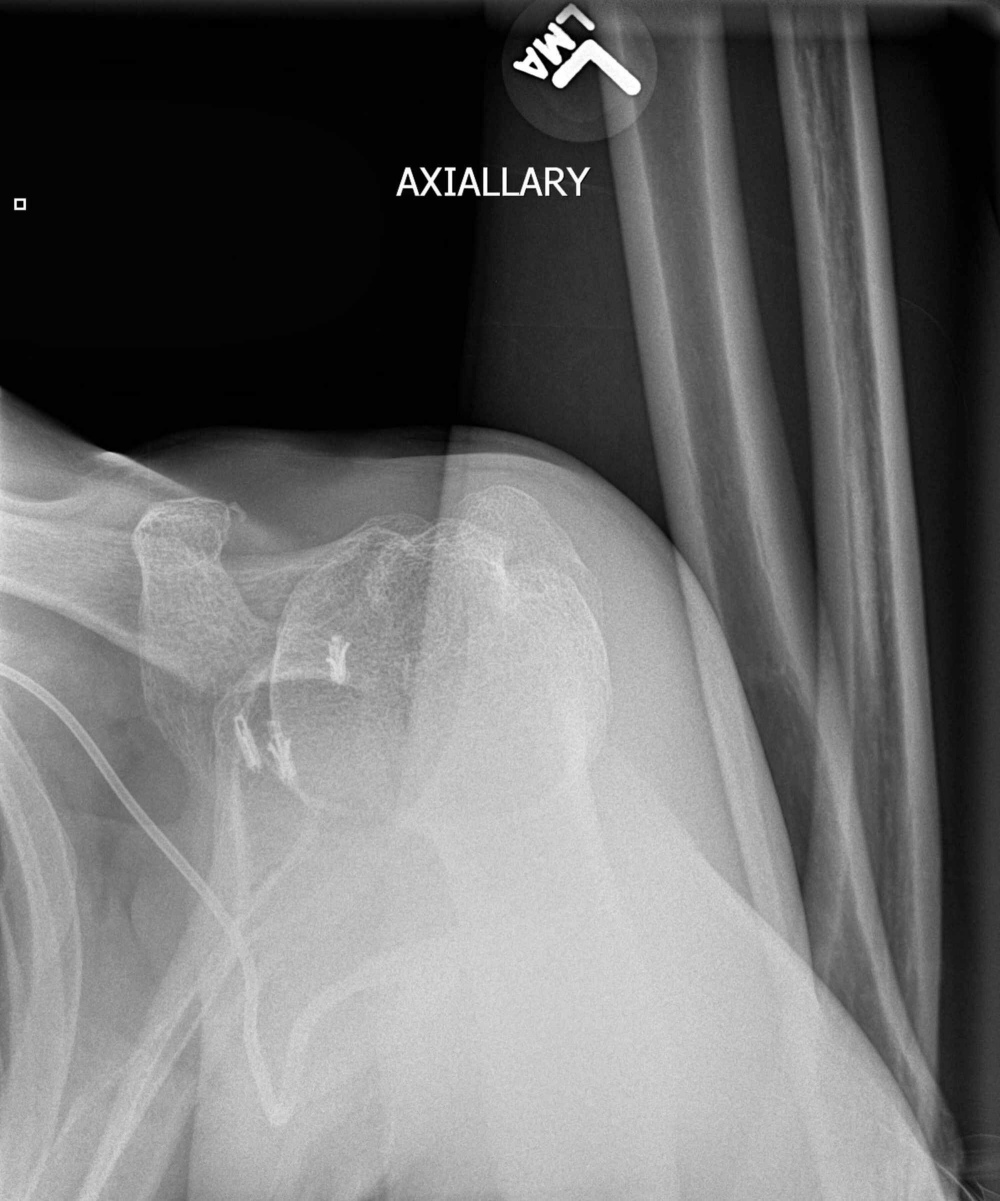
Limited axillary view radiograph of the shoulder showing posteroinferior subluxation of the humeral head
